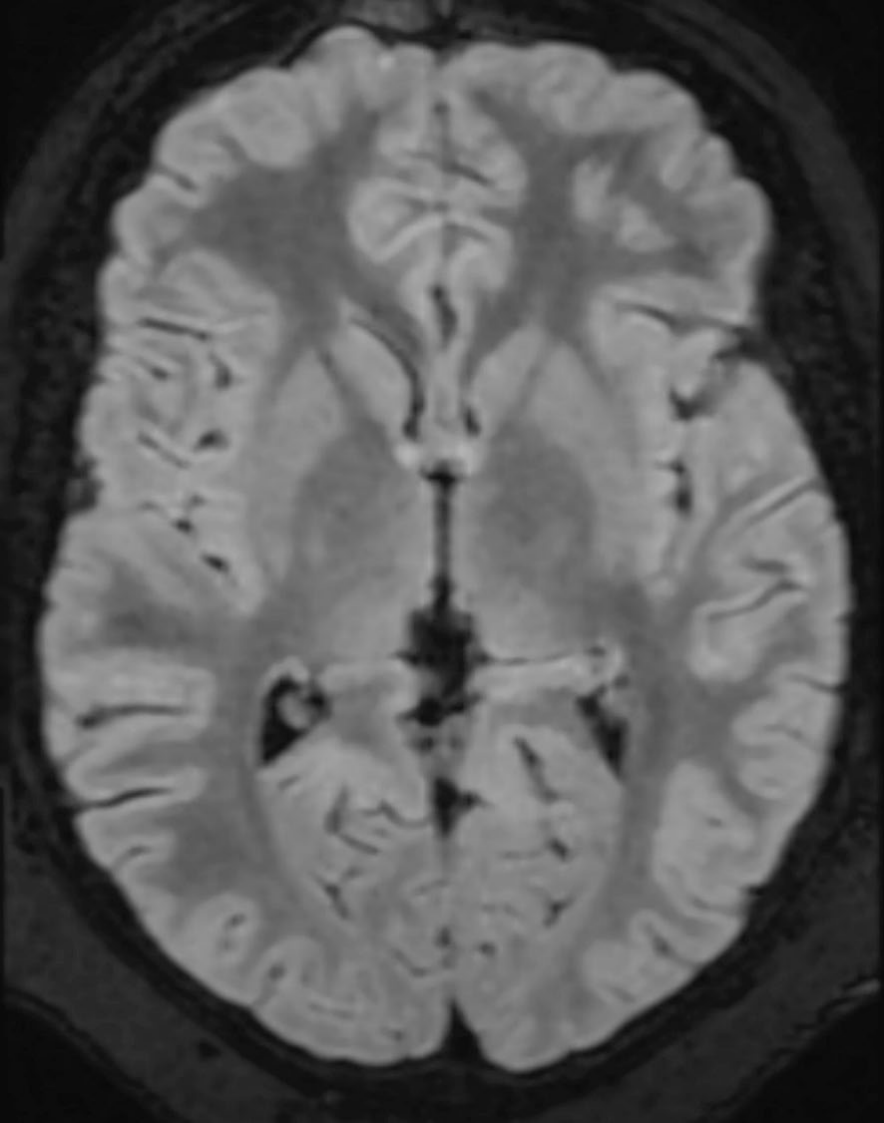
Axial MRI section at the thalamic-hypothalamic level in the 3D FLAIR sequence
